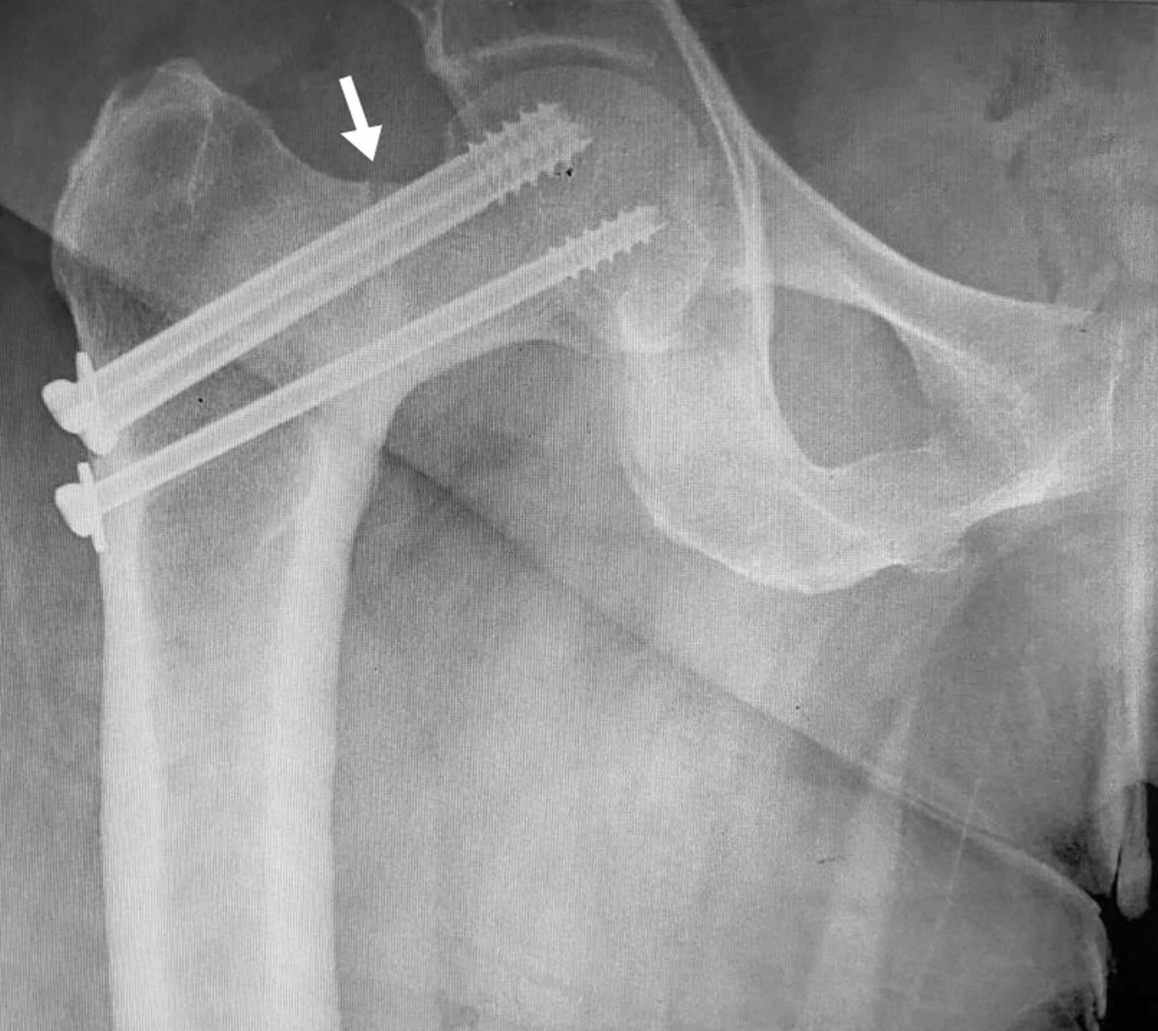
Anteroposterior right hip radiograph with radiolucent line along superolateral aspect likely tension type stress femoral neck fracture (white arrow).
